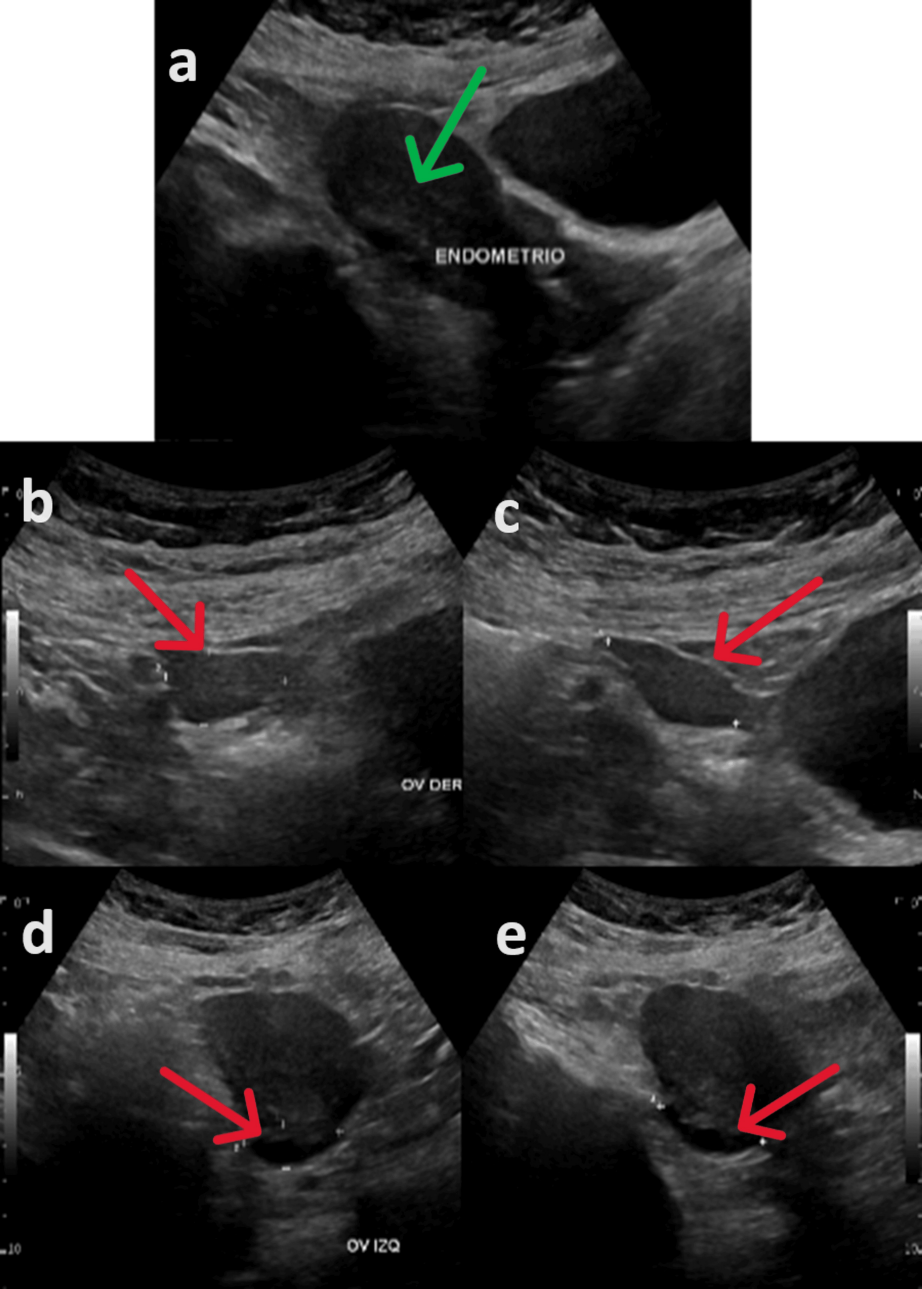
Gynecologic ultrasound showing uterus, right and left ovaries with no significant abnormalities. The images show the uterus, with green arrow pointing to the endometrium (a), the right ovary (b, c), and the left ovary, with red arrows pointing towards the ovary (d, e).
